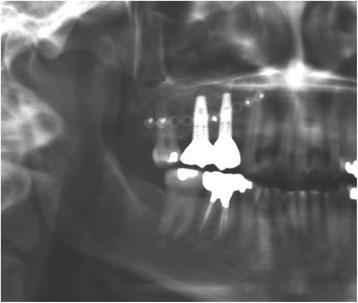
Orthopantomograph 2 years after implant placement
